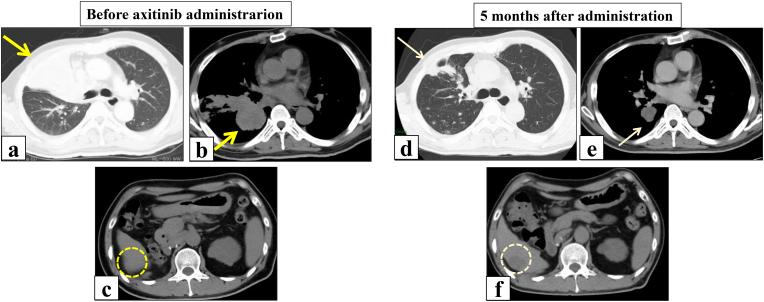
CT findings before and 5 months after administration of avelumab plus axitinib. CT shows multiple lung metastases, right pleural infiltration, pulmonary artery and vein infiltration, and right upper lobe atelectasis due to hilar lymph node enlargement (a,b). Re-expansion of the collapsed lung with shrinkage of the hilar lymph node enlargement and lung metastasis shrinkage after treatment (d,e). Liver metastasis in S6 is also seen before the treatment (c), and it has shrunk after treatment (f).
